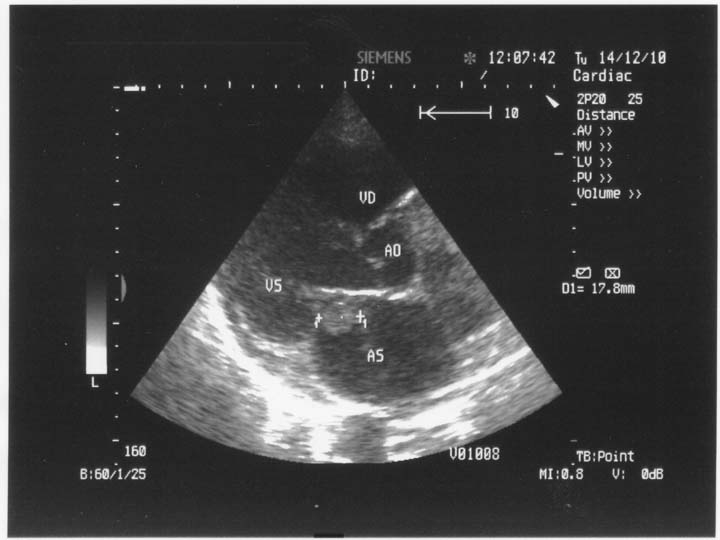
2D Transthoracic echocardiography. Parasternal long axis-view. Left intraatrial thrombus reaching the mitral annular plane. AS=left atrium, VS=left ventricle, VD=right ventricle, Ao=aorta.
